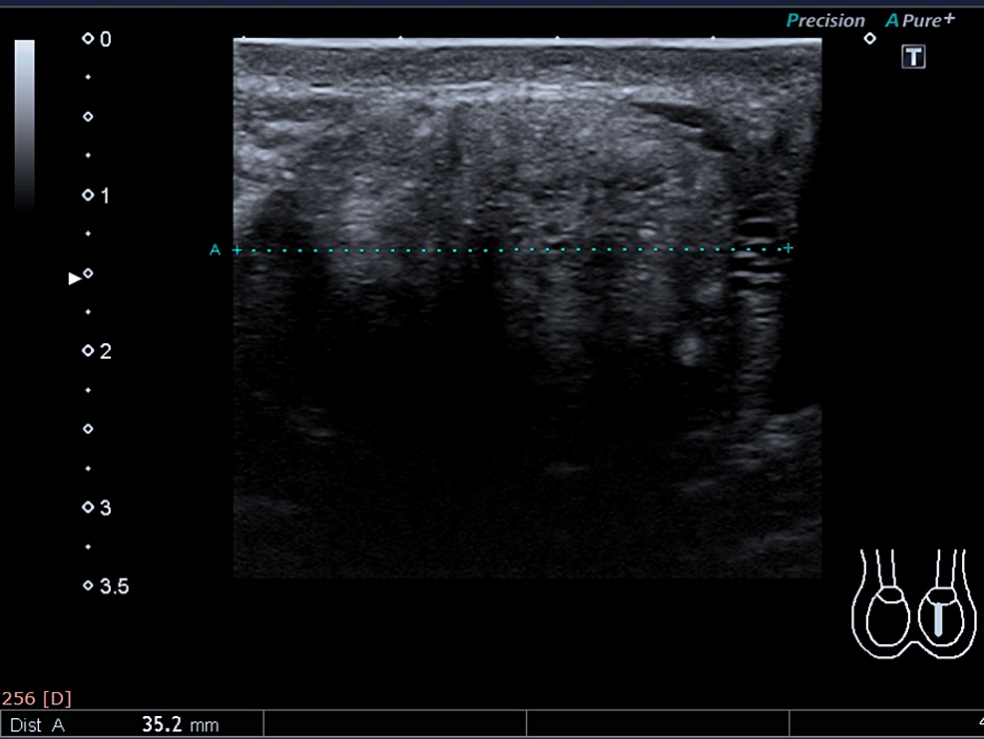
Pre-treatment scrotal ultrasound.
